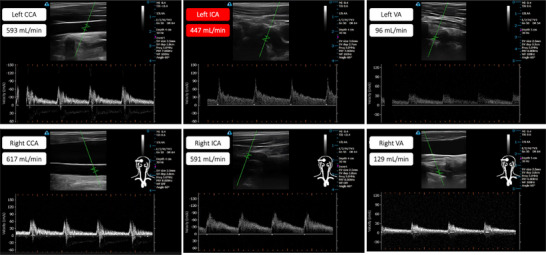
Duplex ultrasound images highlighting partial recovery of anterior and posterior extracranial blood flow in the patient. CCA, common carotid artery; ICA, internal carotid artery; VA, vertebral artery. Red shading highlights (incomplete) recovery perfusion in the (left) dissected ICA.
